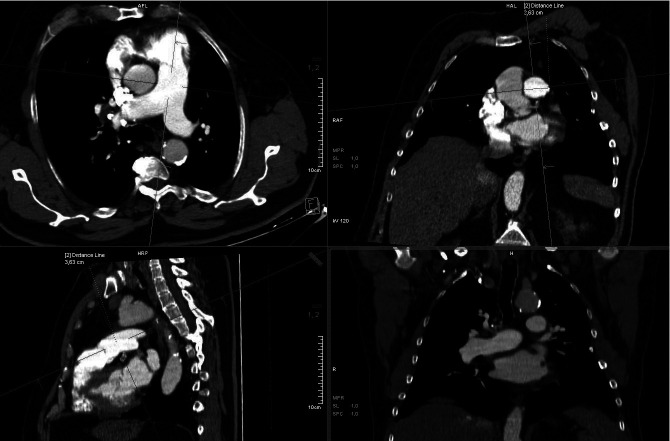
Measurement of the pulmonary artery and aorta width on the same slice of the computed tomography scan.
